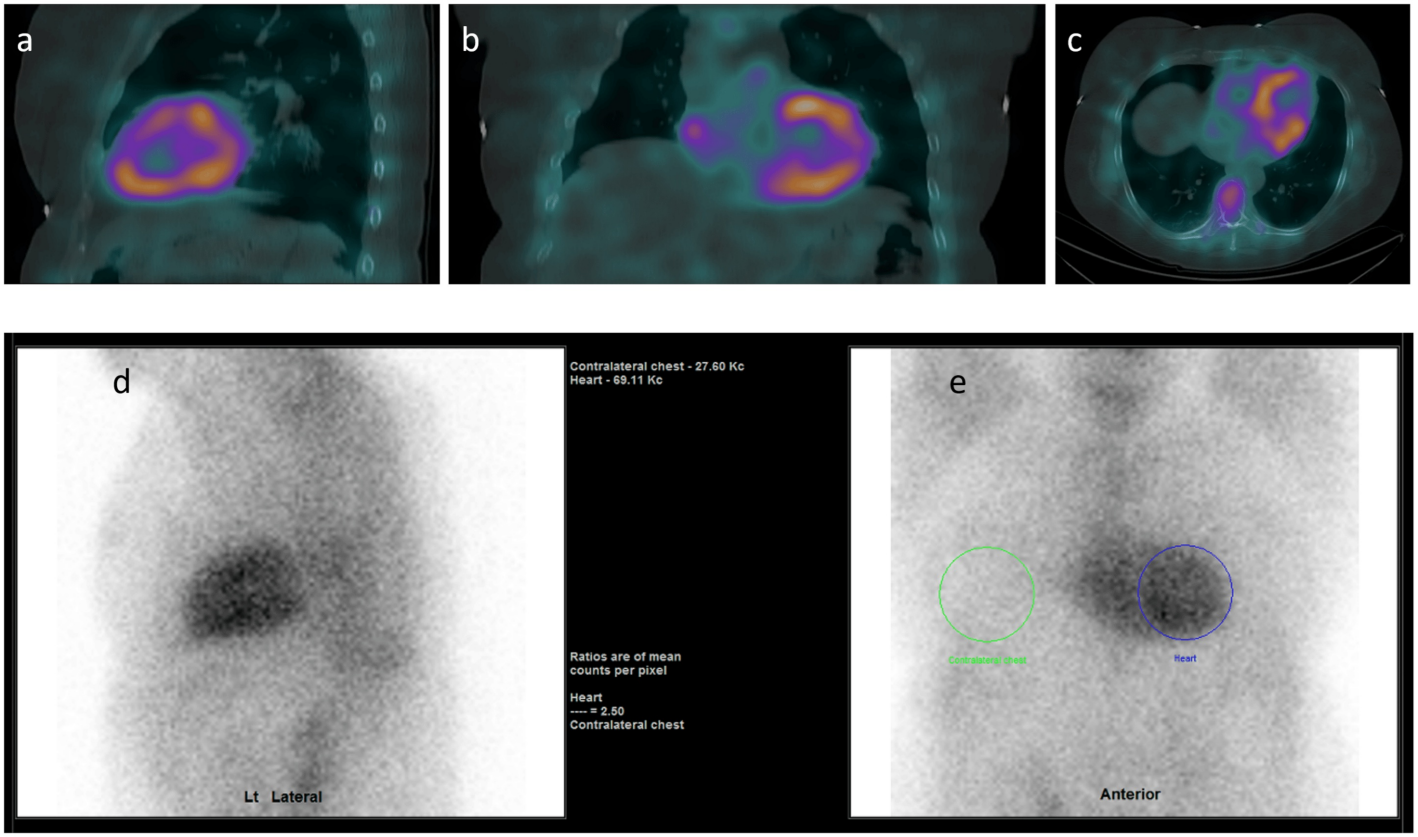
Nuclear Cardiac Scintigraphy Tc-99m pyrophosphate cardiac scintigraphy (PYP) showing coloured left ventricular myocardial avidity (a-d) with cardiothoracic standardised uptake value ratio >1.5 (e) consistent with cardiac amyloidosis [10]. *Tc-99m: Technetium-99m.
